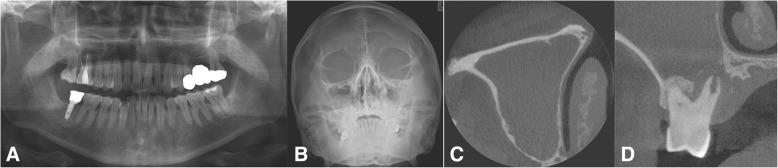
A representative case of odontogenic maxillary sinusitis originating from an apical lesion in the right upper second molar. Preoperative panoramic (a), Water’s (b), axial cone-beam CT scan (c), and coronal cone-beam CT scan (d) views
